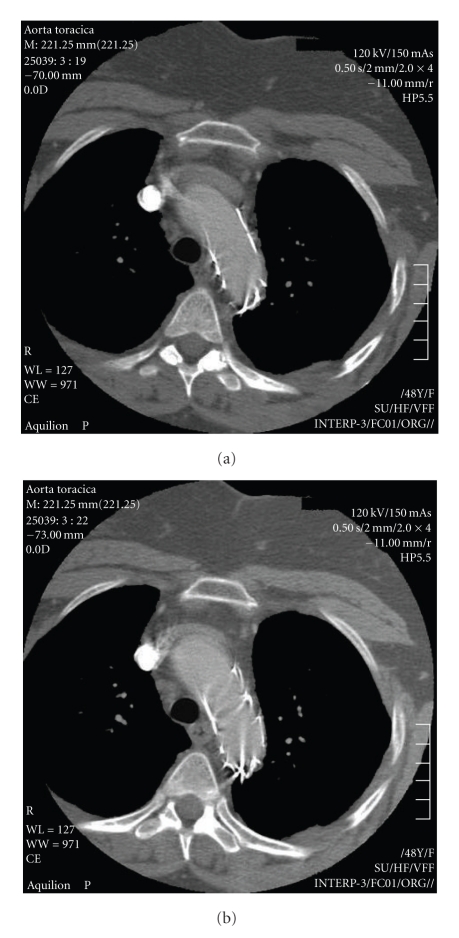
Angio-CT scan showing follow-up one year after intervention.
